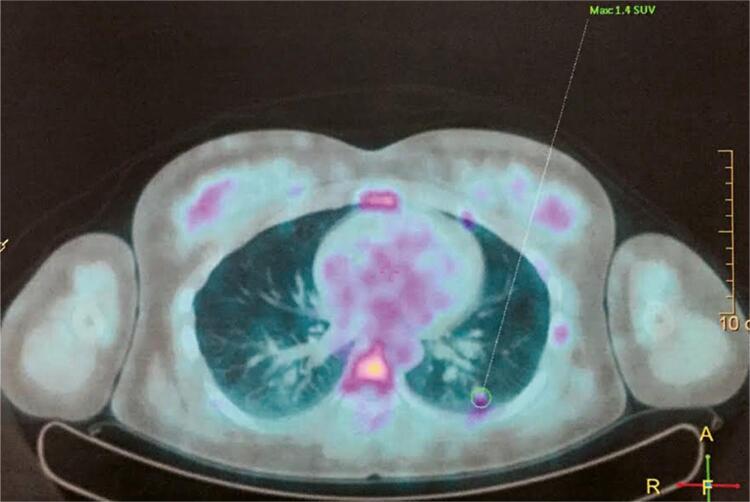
A suspicious lung nodule investigated with 18F-FDG PET-CT showing discrete glucose uptake (SUV of 1.4).
